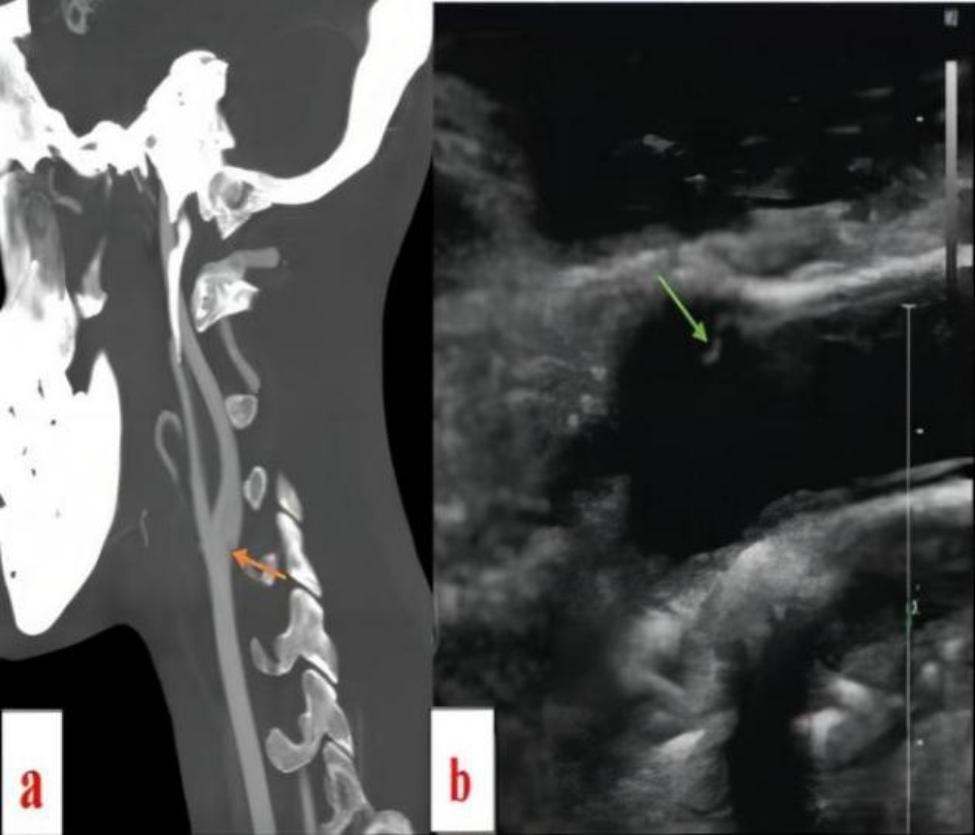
a CTA showed CaW (orange arrow), b Carotid ultrasound showed CaW (green arrow)
